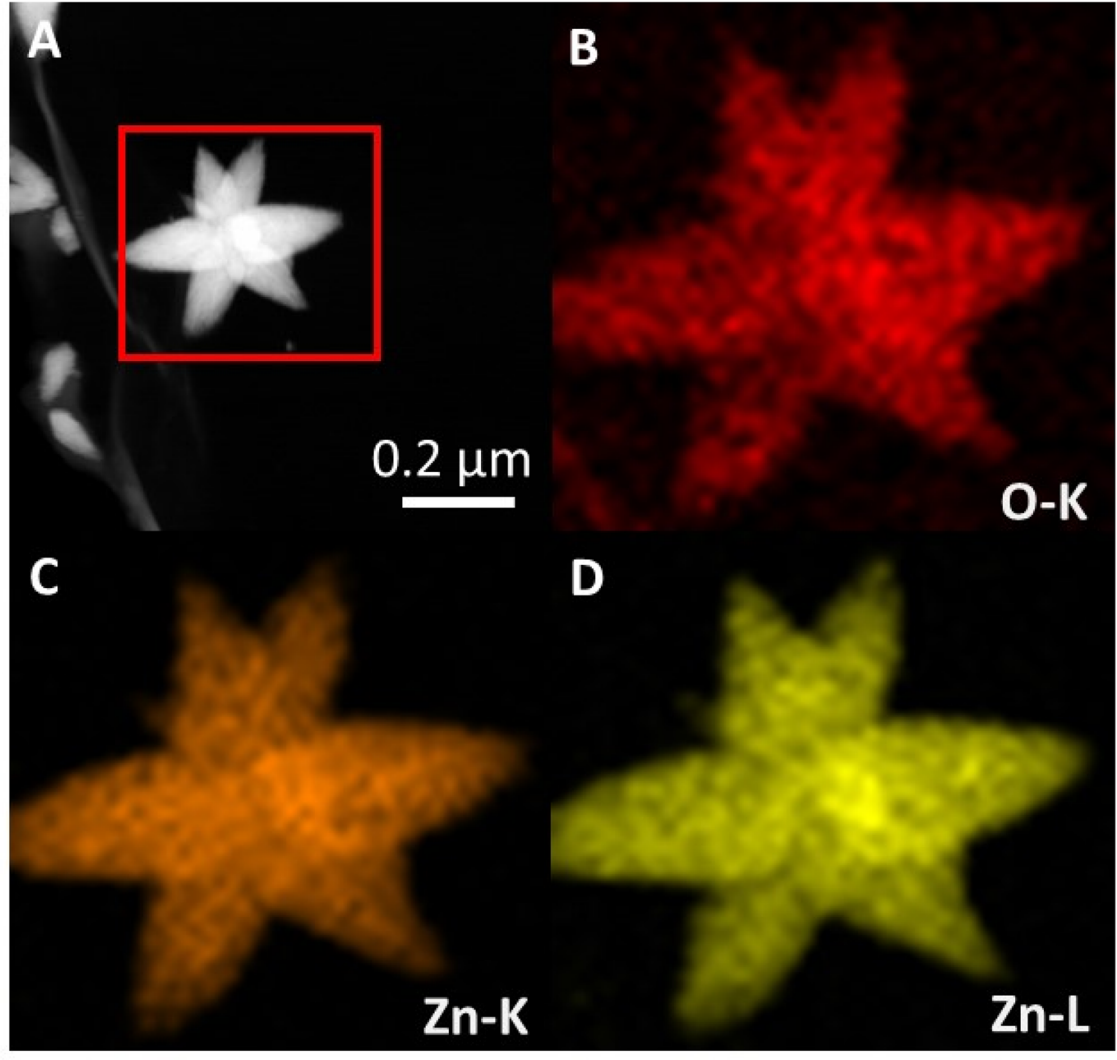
STEM images of the ZnO star (A) with corresponding elemental maps of characteristic X-ray: O K-line (B), Zn K-line (C) and Zn L-line (D). EDX maps were acquired from the region marked by a red square.
